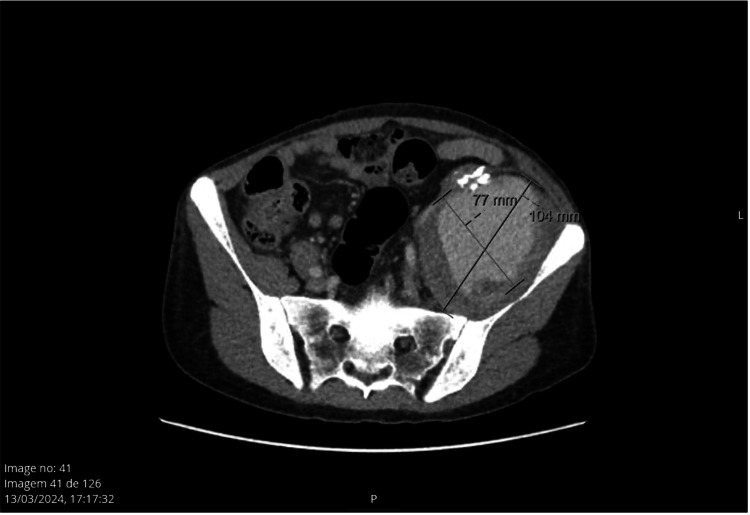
CT scan showing a mass of 77 x 104 mm in the left iliac fossa.
